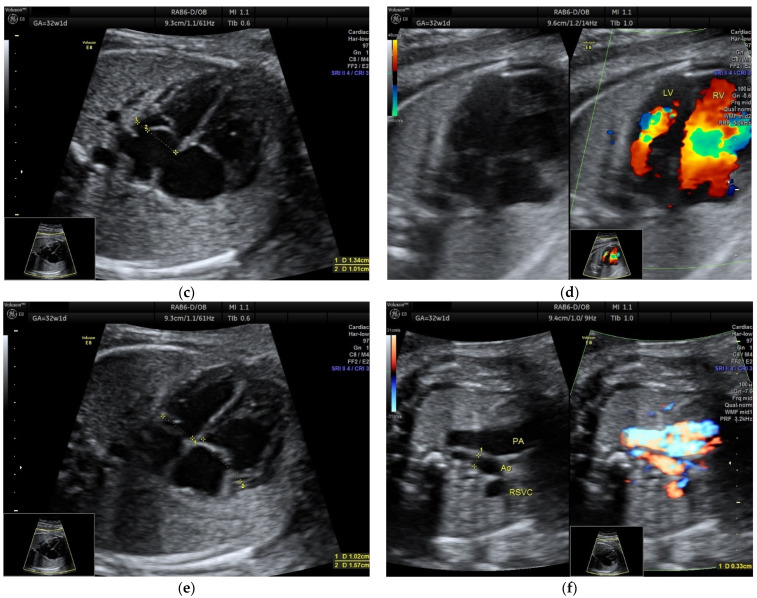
The second case is a 24-year-old G1P0, without priors, that presented at 21w1d for her second trimester screening scan. An atrial septal aneurysm was also demonstrated (ASE index = 0.70) (a), with normal LV inflow (b). At 32w1d, the foramen ovale flap was making a cyclical contact with the mitral valve, almost covering it (ASE index = 0.75) (c), and significantly reducing the LV inflow (d). This diminished preload had led to a thinner LV (cardiac chamber asymmetry, RV/LV = 1.53) (e). The aortic arch had an anterograde flow but was significantly smaller than in the second trimester (aortic isthmus Z-score = −2.03) (f), supporting further evidence of reduced LV output (Video S1). No PACs were noted until delivery. Due to the suspected aortic arch hypoplasia, the patient delivered in a third-level maternity, where neonatal cardiovascular surgery was available on site. A baby boy weighing 3690 g was delivered by C-section at 39 w, with good postnatal adaptation. The transthoracic echocardiography performed in the early neonatal period showed normally sized LV and aortic arch, while the foramen ovale closed incompletely, with a double interatrial communication. There was also a suspicion of aortic valve malformation, which was not confirmed at the 6 months follow-up, when only a 2.5 mm patent foramen ovale was present. ASE index, atrial septal excursion index; LV, left ventricle; RV, right ventricle; PAC, premature atrial contraction; PA, pulmonary artery; Ao, aorta; RSVC, right superior vena cava.
